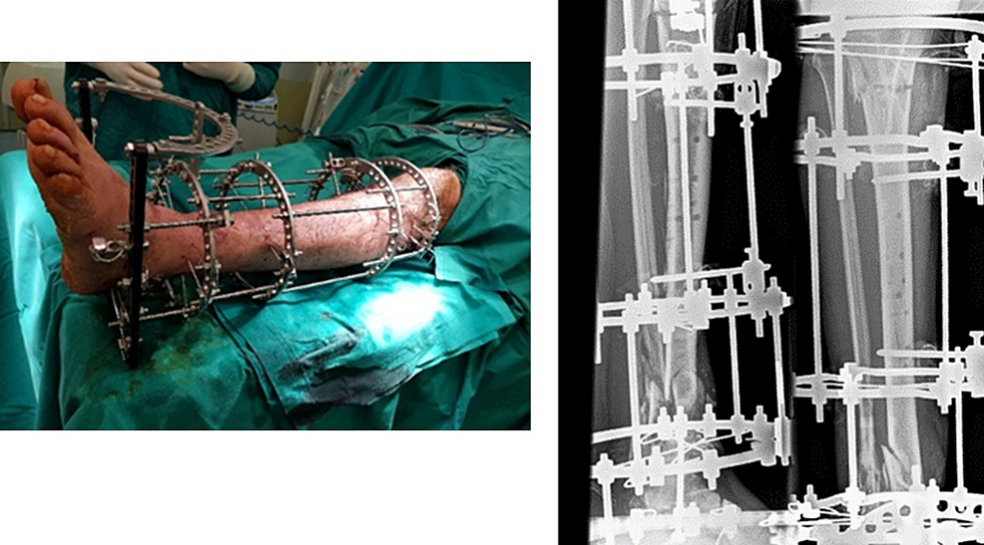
Intraoperative image and post-operative x-rays of the Ilizarov technique.
